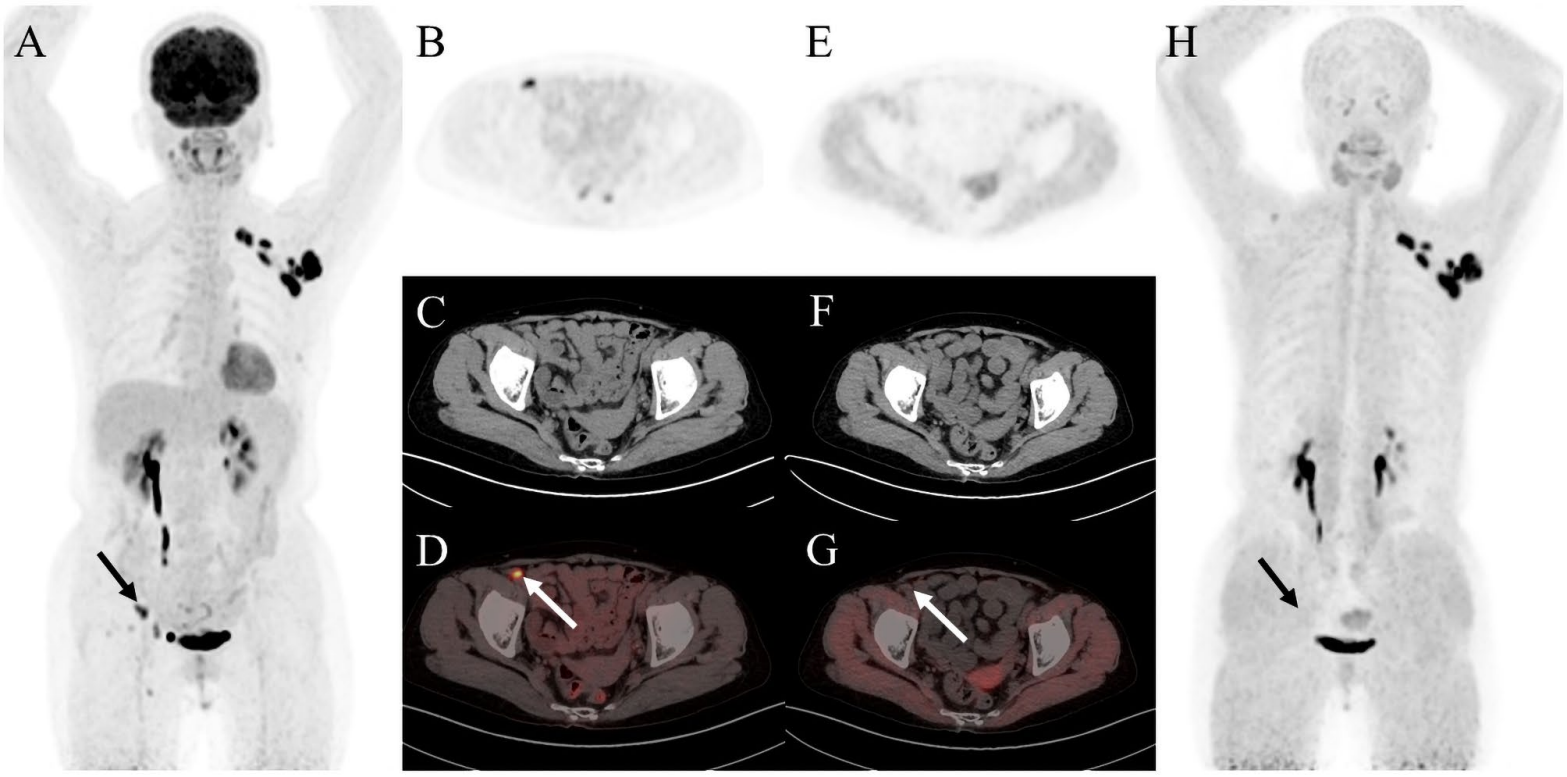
A 68-year-old woman was admitted to our hospital due to the discovery of a left axillary mass for over 10 days. The ^18^F-FDG PET/CT and ^68^Ga-FAPI PET/CT were performed for initial assessment. The MIP image(A) and the axial views of ^18^F-FDG PET/CT (B: PET image; C: CT scan; D: PET/CT fused image) showed suspicious lymph nodes in the right external iliac region (solid arrow, SUV_max_, 8.8). However, it showed no corresponding uptake on ^68^Ga-FAPI PET/CT (E: PET image; F: CT scan; G: PET/CT fused image; H: MIP). Subsequently, the patient underwent lymph node puncture and pathological results revealed a small number of lymphocytes
